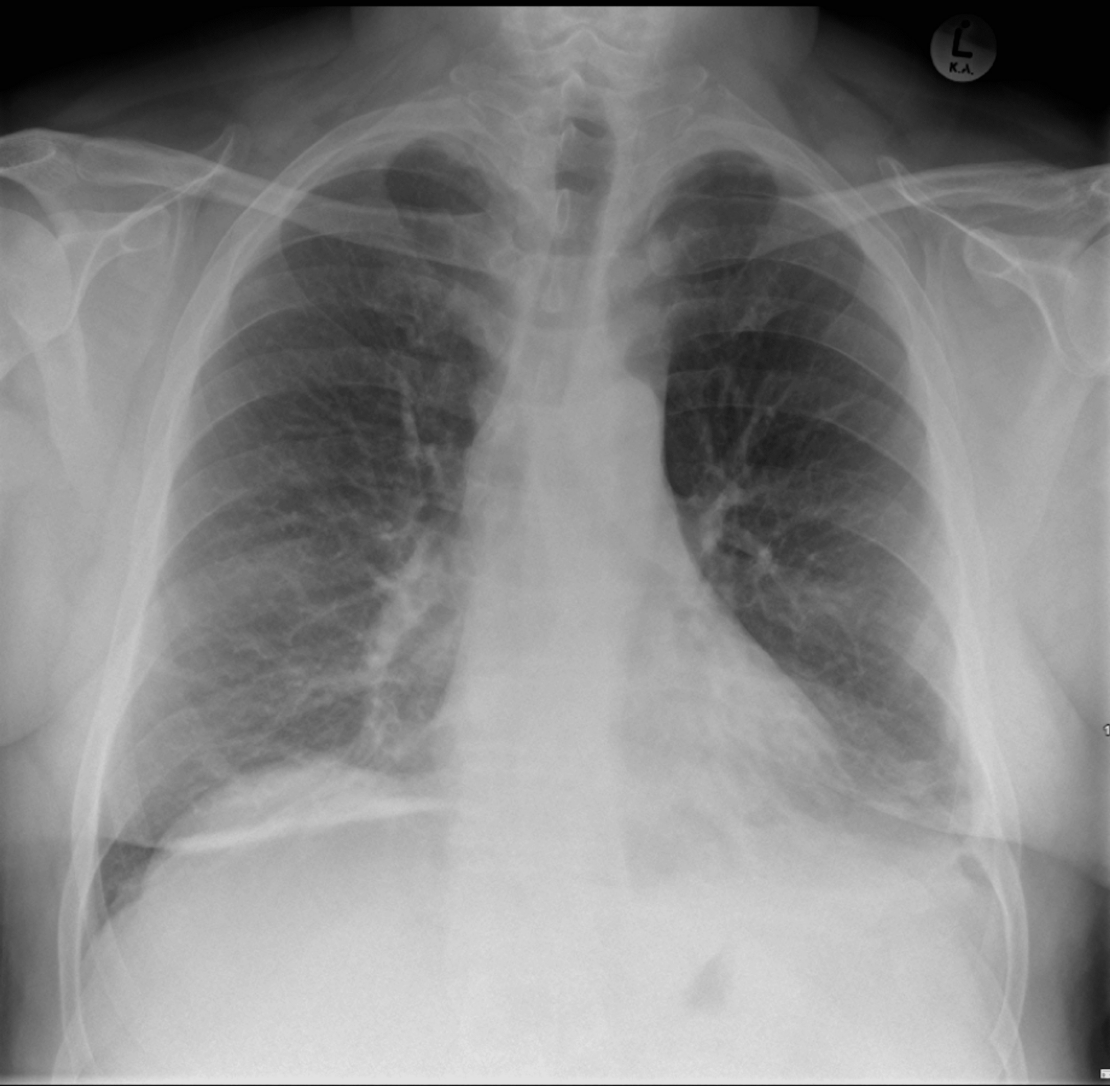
Chest X-ray from initial presentation with lower respiratory tract infection symptoms
